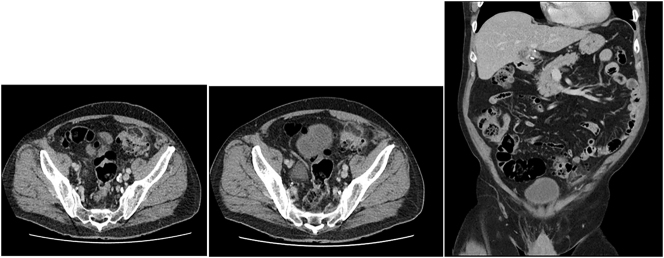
Abdominal CT scans – axial (A and B) and coronal sectional plane (C): Extended diverticulosis and appendicitis epiploicae in the immediate subperitoneal region of the left lower abdomen with an oval fat isodense structure in the region of the sigmoid colon starting from the anterior wall with surrounding inflammatory inhibition and pronounced intestinal wall.
